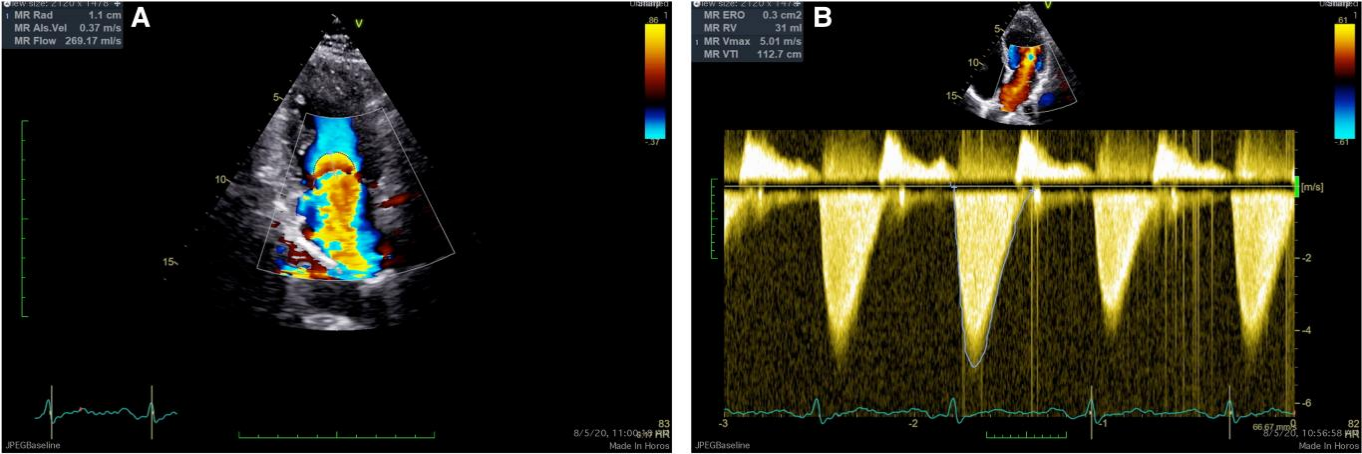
Recurrence of severe mitral regurgitation with validated quantification in transthoracic echocardiogram. (*A*) Pisa mitral regurgitation 1.1 cm indicating severe regurgitation. (*B*) Effective regurgitant orifice area and regurgitant volume measured with continuity method. Note that the triangular shaped envelope of mitral regurgitation indicates acute severe mitral regurgitation and that the effective regurgitant orifice area and regurgitant volume could be underestimated since the continuity equation assumes a more uniform flow.
